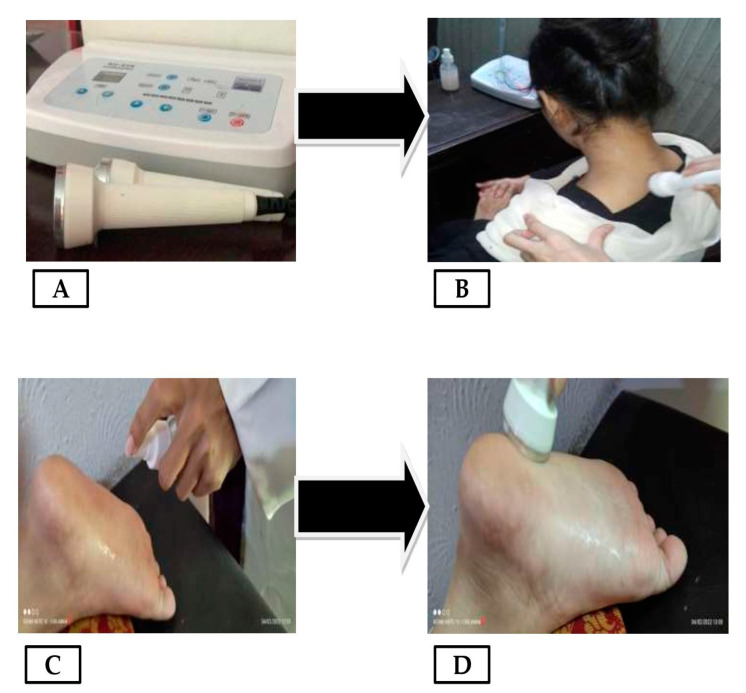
(A) Phonophoresis (ultrasound) for the treatment of sport injuries, (B) Phonophoresis on a patient with neck pain after applying 4 pumps of DK gel, (C) Applying 4 pumps of DK gel on a patient foot, (D) Phonophoresis on a patient foot.
